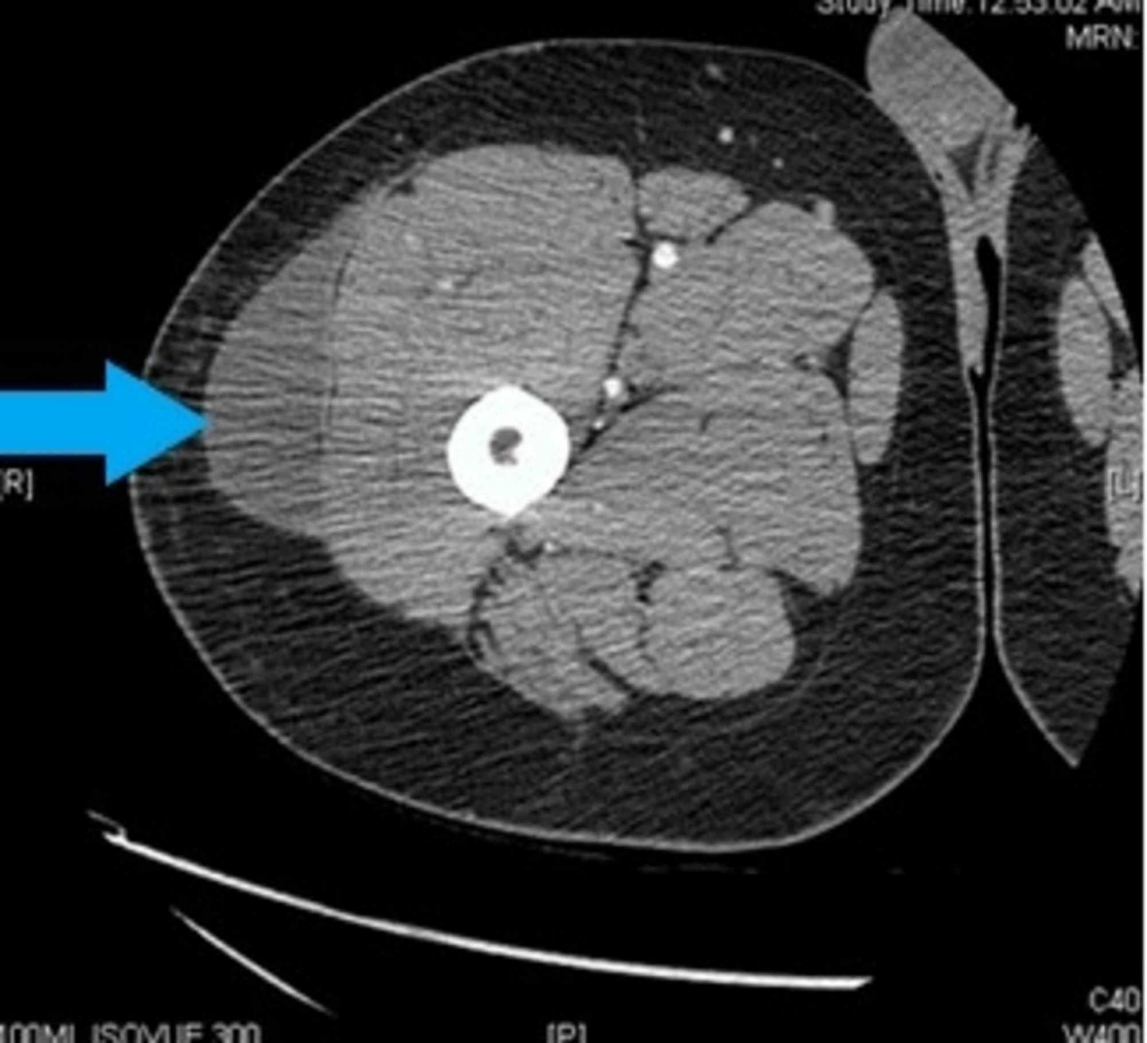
CT with IV contrast right lower extremity (axial view) showing a fluid collection within the deep aspect of the subcutaneous soft tissues
